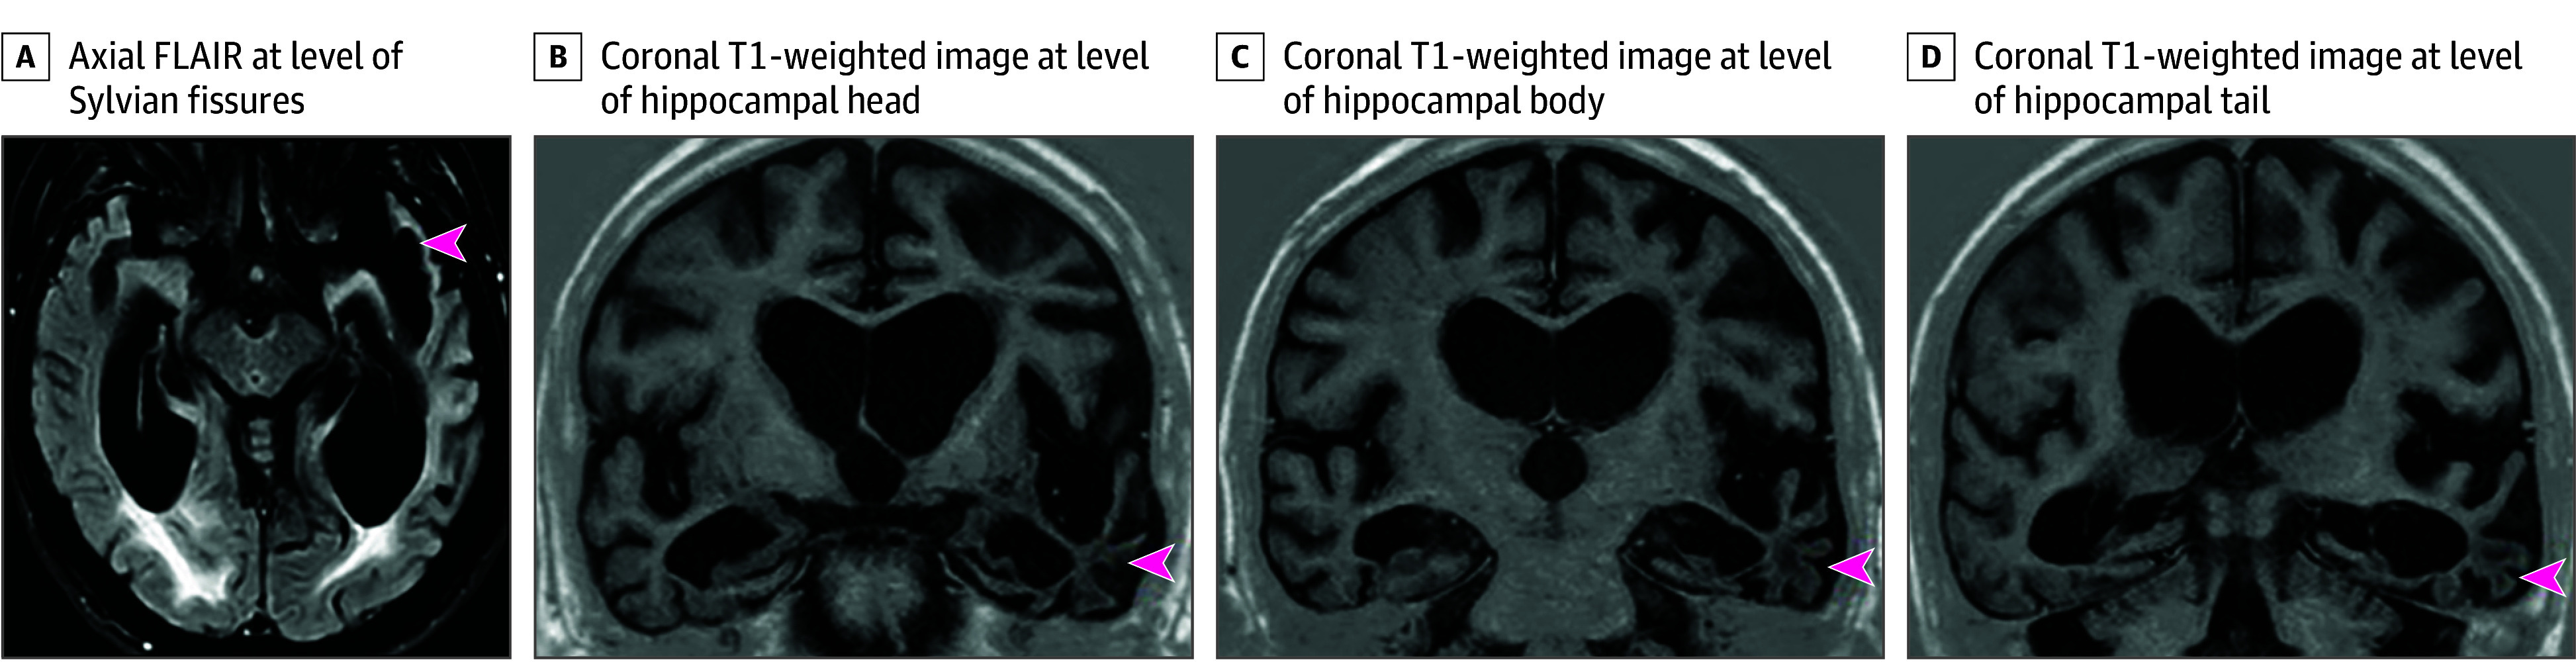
Magnetic Resonance Head Imaging Performed in the Last Year of Life Axial fluid-attenuated inversion recovery (FLAIR) (A) shows asymmetrical widening of the sylvian fissures (red arrowhead), which is more apparent on the left than the right. Coronal T1-weighted images at the level of the head (B), body (C), and tail (D) of the hippocampus show severe mesial temporal lobe volume loss bilaterally, again affecting the left side more than the right (arrowheads).
